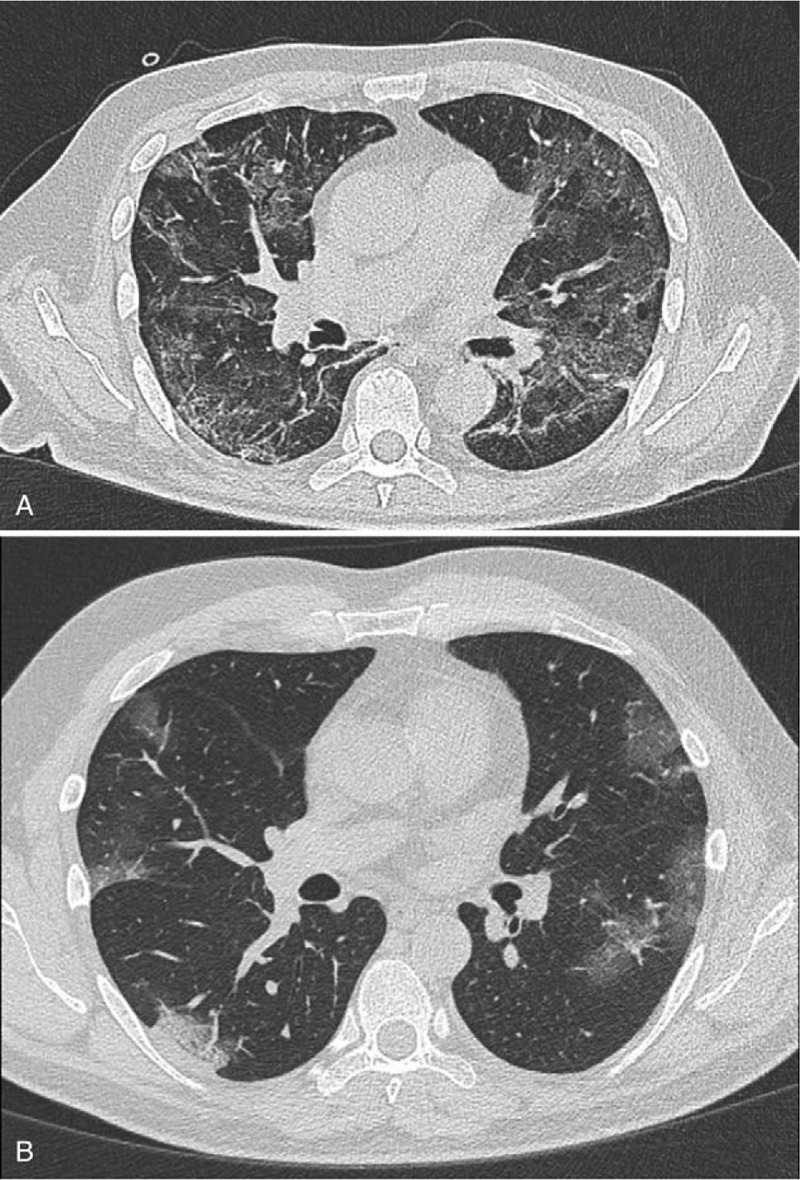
A-B. Chest Computed Tomography scan showing bilateral ground glass opacities with multilobar involvement.
